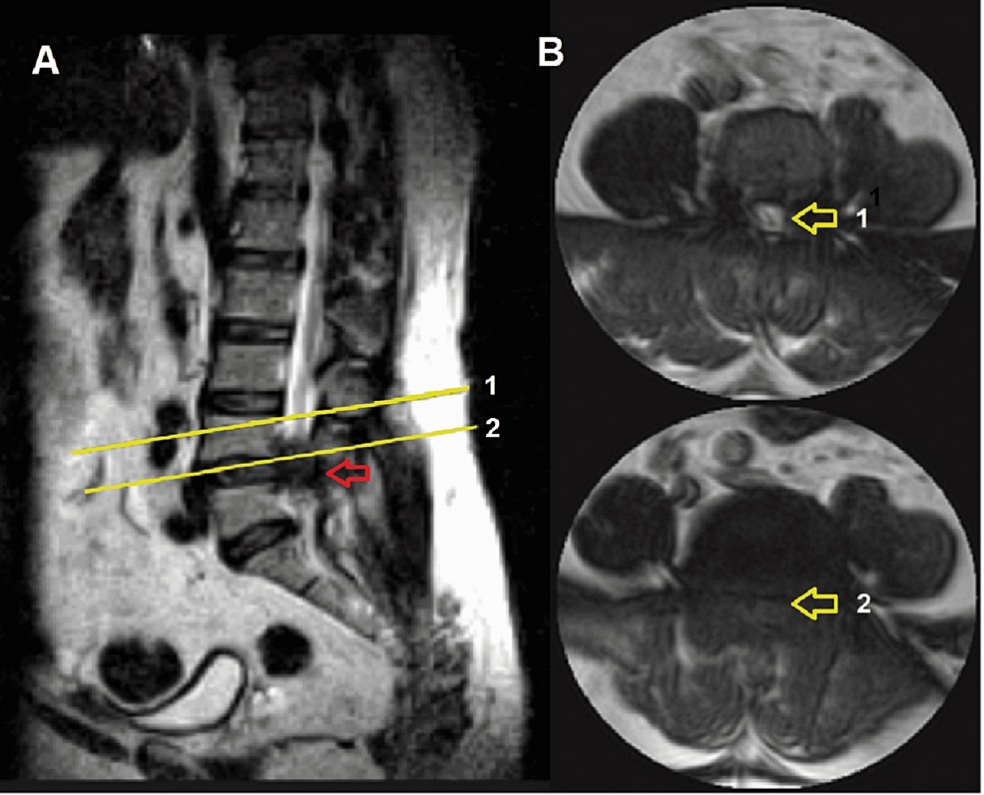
MRI of the lumbar spine. Sagittal (A) and axial (B) views in T2 sequence. The red arrow shows the tophus as a hypointense mass obliterating the spinal canal. The yellow arrows indicate: 1: axial section above the level of the lesion showing a normal spinal canal. 2: axial section at the level of the lesion showing complete obliteration of the spinal canal.
